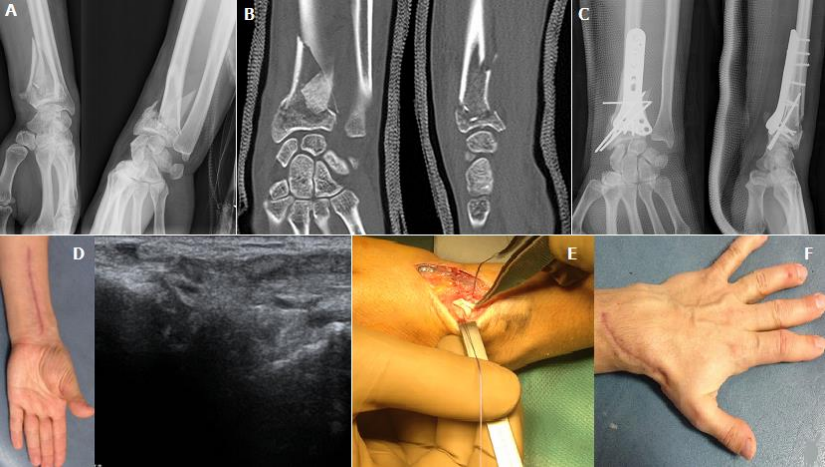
radiographie standard du poignet gauche montrant une fracture épiphysométaphysaire communitive déplacée de l’extrémité inférieure du radius; (B) scanner du poignet gauche objectivant la complexité de la fracture du radius distal atteignant la surface articulaire; (C) radiographie post opératoire de contrôle. Ostéosynthèse par plaque antérieure visée + broches avec une bonne réduction fracturaire; (D) aspect clinique et échographique de la rupture secondaire du long extenseur du pouce; (E) Image péropératoire. Aspect effiloché du tendon accroché dans un important cal osseux; (F) résultat clinique à j60 post opéraoire
